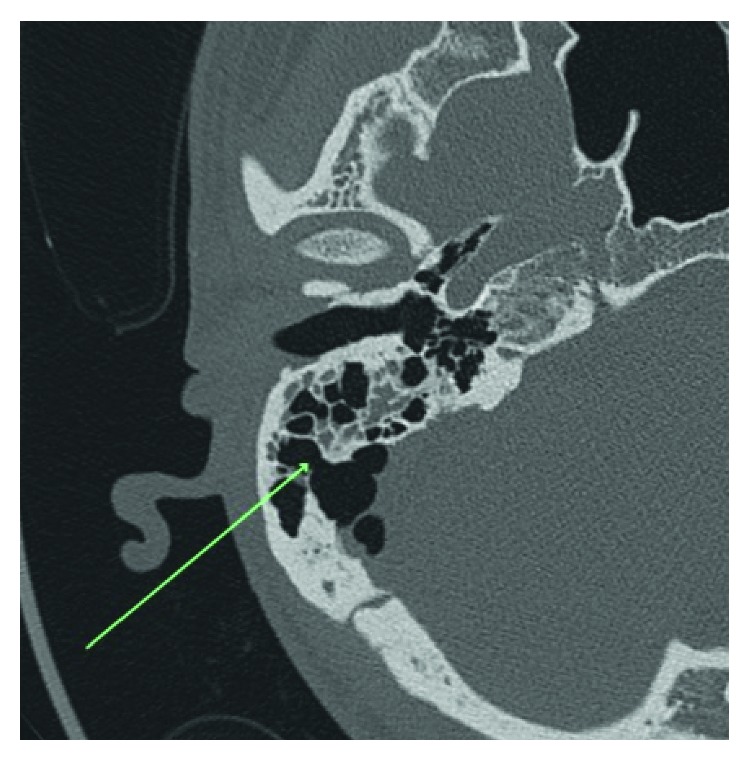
Axial view of CT head demonstrating mastoid defect and pneumocephalus.
